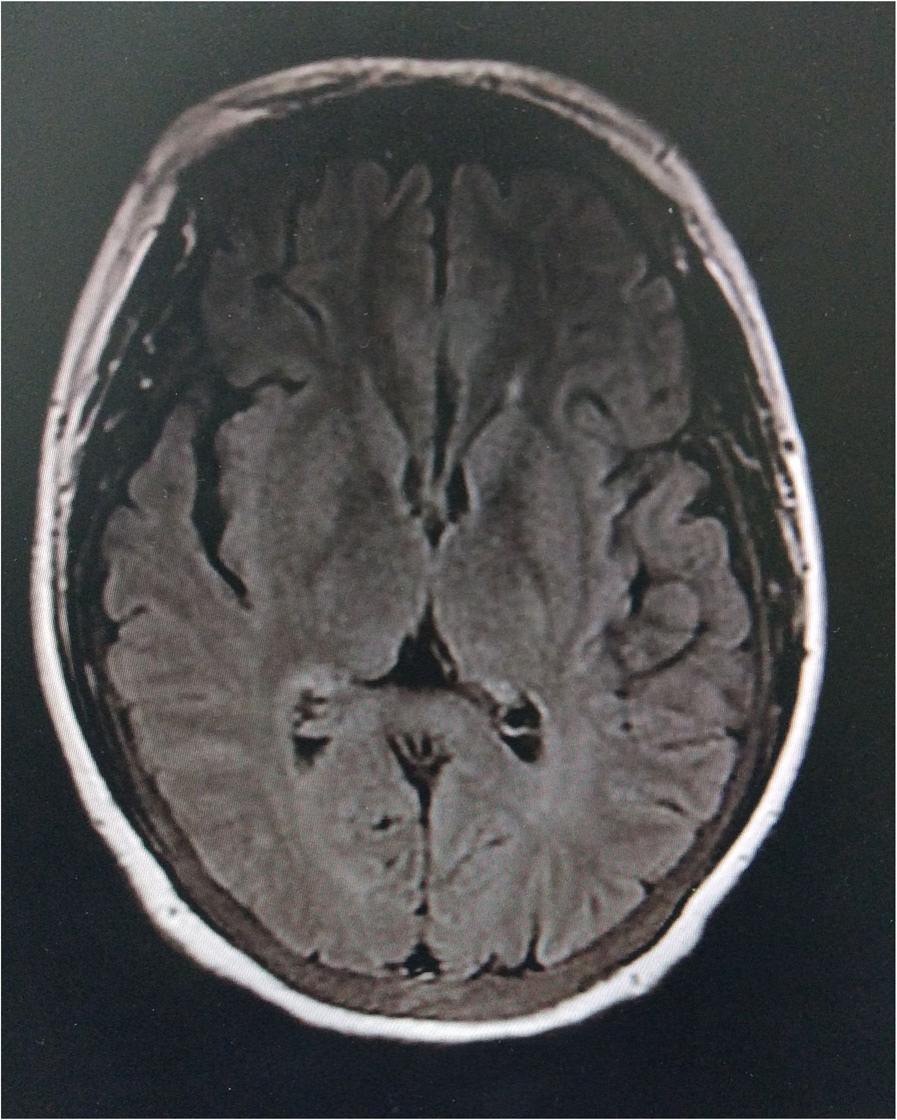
Follow-up MRI scan obtained after 1 month. T2FLAIR showed almost complete resolution of the lesions with a slightly hyperintense signal
